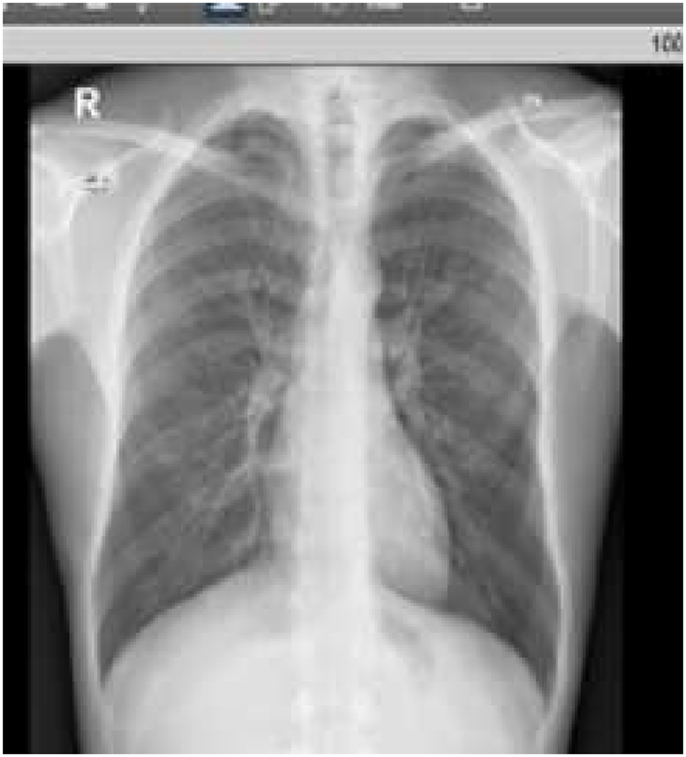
Chest x-ray PA, March 2021: the left upper lobe opacity resolved.
